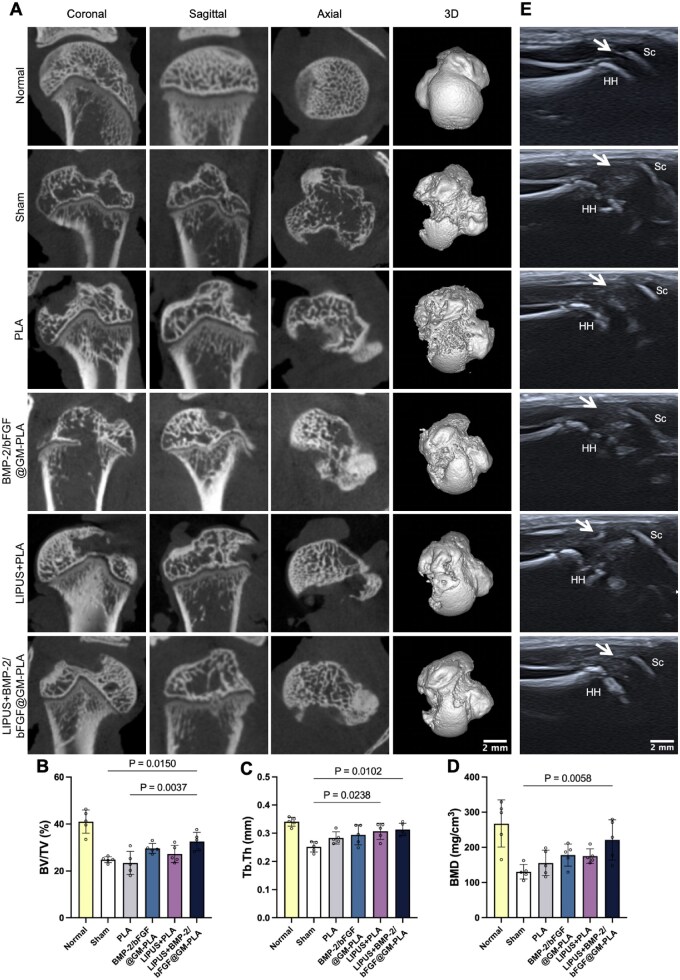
Radiologic evaluation of the bone tunnel and repaired tendon. (A) Representative Micro-CT and 3D reconstructed images of the humerus at 6 weeks postoperatively. (B–D) Analysis of the CT-based bone tissue structural parameters of the footprint and the bone tunnel area: (B) bone volume/tissue volume (BV/TV), (C) trabecular thickness (Tb.Th), (D) bone mineral density (BMD) (for panels B–D, *n* = 5, data are expressed as means ± SD, one-way ANOVA, only statistically significant *P*-value is displayed; the normal group served as qualitative reference and was excluded from the *post hoc* pairwise comparisons). (E) Representative high-frequency ultrasound images of the supraspinatus tendon area (HH: head of humerus, Sc: scapula, white arrow: supraspinatus tendon insertion).
